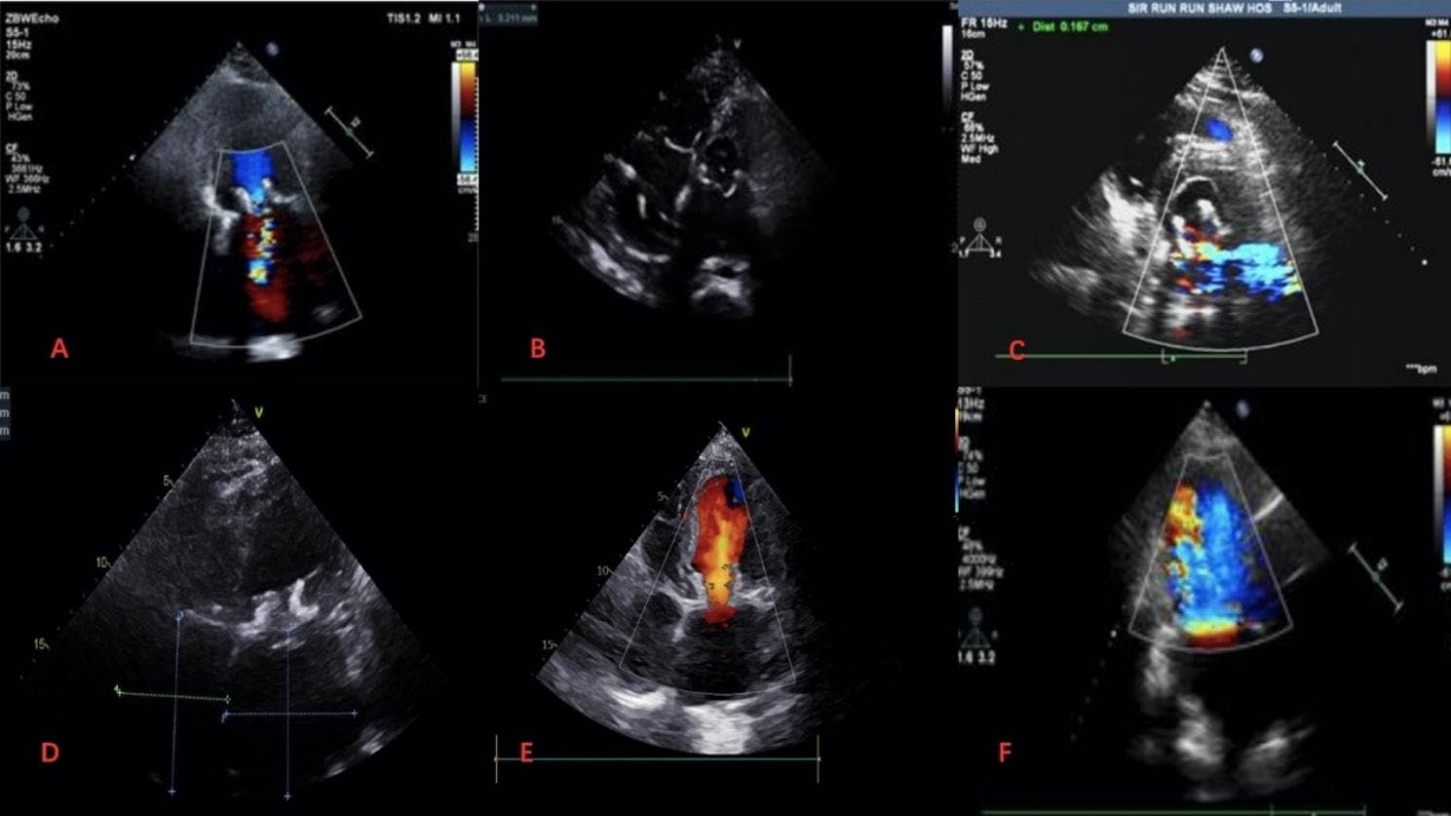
A, B and C were the preoperative echocardiography images of cases 1, 2 and 3, respectively. D, E, and F were the images after J-valve implantation respectively
